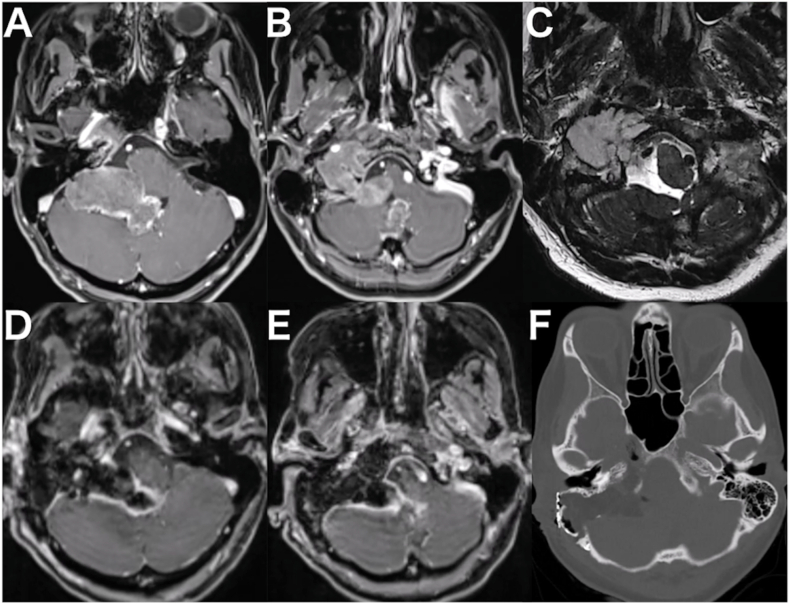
The imaging studies of the case 1 in which right-sided e-TIA was performed. A and B: Preoperative gadolinium enhanced T1-weighted MRI revealing the tumor with contrast enhancement extending from the petrous apex into the cervical and intracranial regions. C: Preoperative T2-weighted MRI demonstrating a hyperintense tumor. D and E: Postoperative Gadolinium enhanced T1-weighted MRI indicating near total resection. F: Postoperative CT scan showing adequate drilling of the petrous bone, including the petrous apex.
